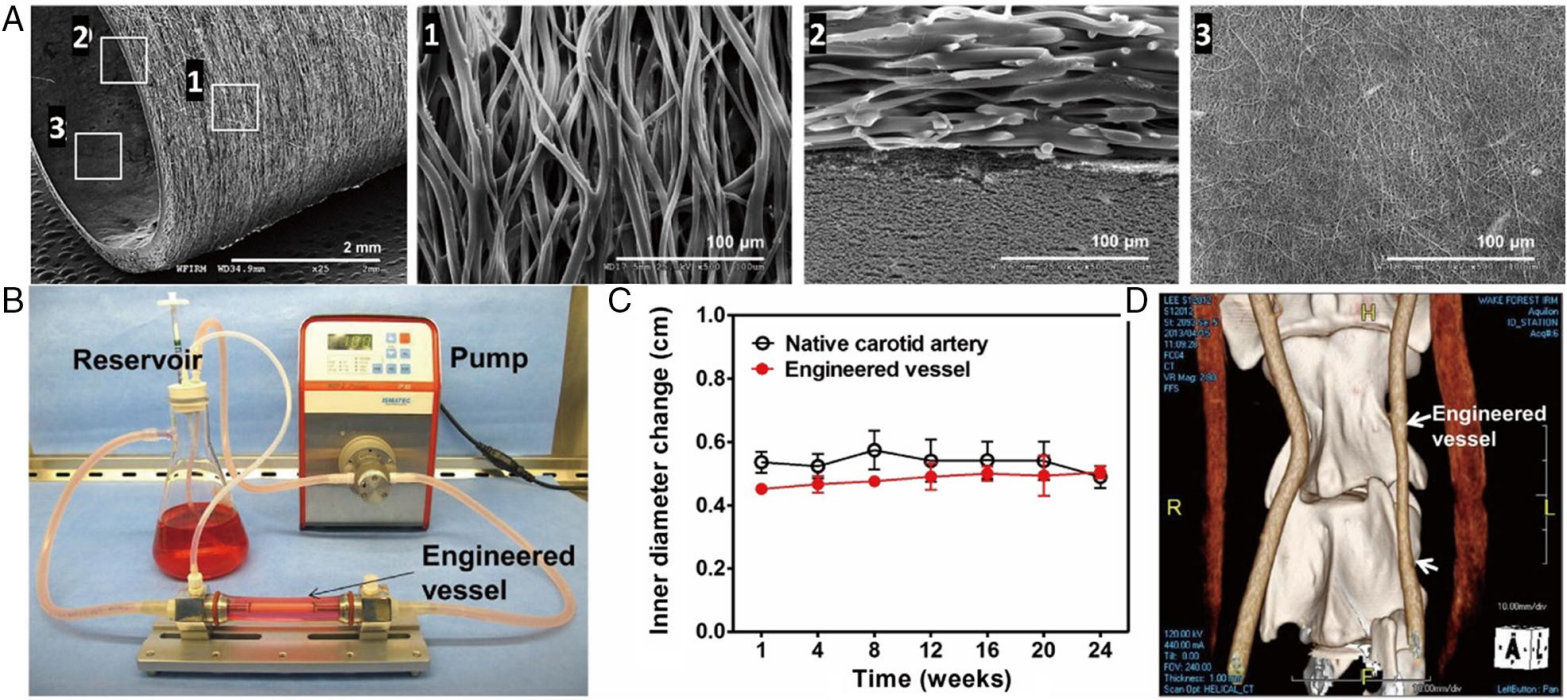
A) SEM images of entire layer, outer layer, cross‐sectional interface and inner layer of the bilayered vascular scaffold. B) Bioreactor setup consisting of a computer‐programmed gear pump, flow reservoir, and the bioreactor housing unit. C) Inner diameter measurement of the transplanted engineered blood vessels revealed a stable lumen caliber for the duration of the 6‐month follow‐up. D) A representative CT scan shows the absence of an aneurysm along the entire length of the transplanted engineered blood vessel (arrows). A–D) Reproduced with permission.^[^
